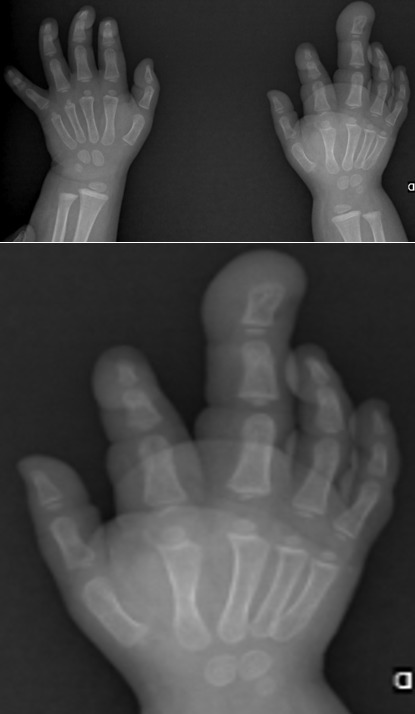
Radiographie standard radiographie des mains montrant une macrodactylie du 3^ème^ rayon de la main droite. Noter l'opacité des parties molles palmaires
